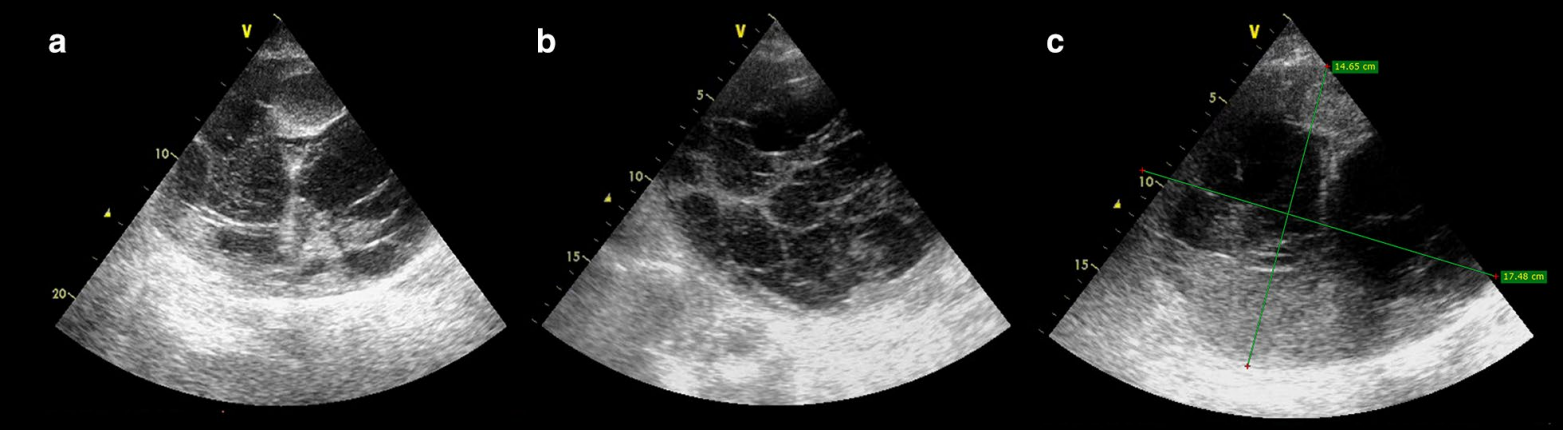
Abdominal ultrasound from the left coronal view showing a complex cystic mass (a and b) with multiple internal septations, measuring approximately 18 cm × 15 mm (c), highly suggestive of a large rectus sheath hematoma (RSH)
